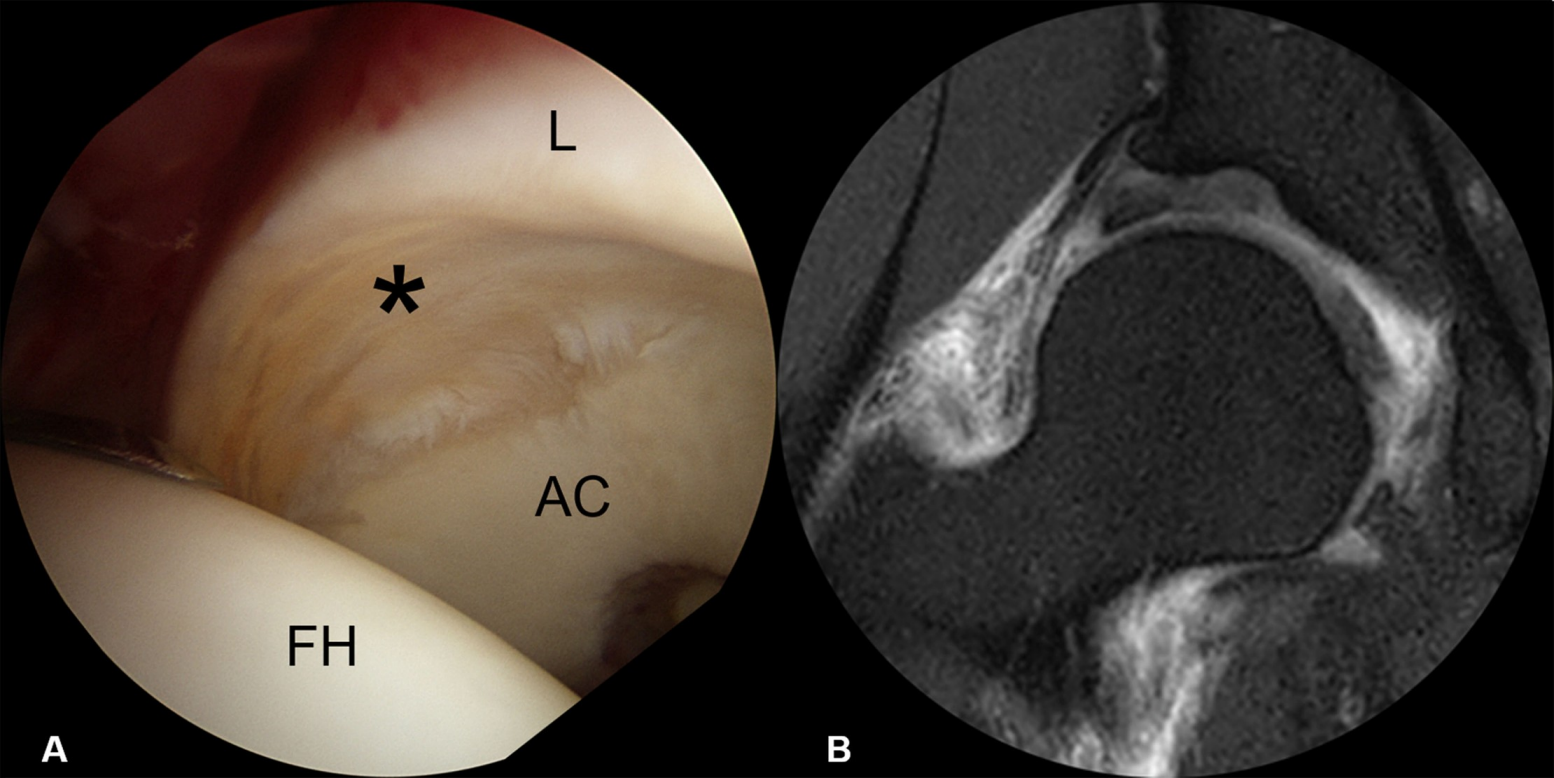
Arthroscopic findings and MRI features of grade 1 labral tears. (A) Arthroscopy showing partial delamination with fraying at CLJ, with (B) MRI showing no definite labral pathology. (L, labrum; FH, femoral head; AC, acetabular cartilage).
